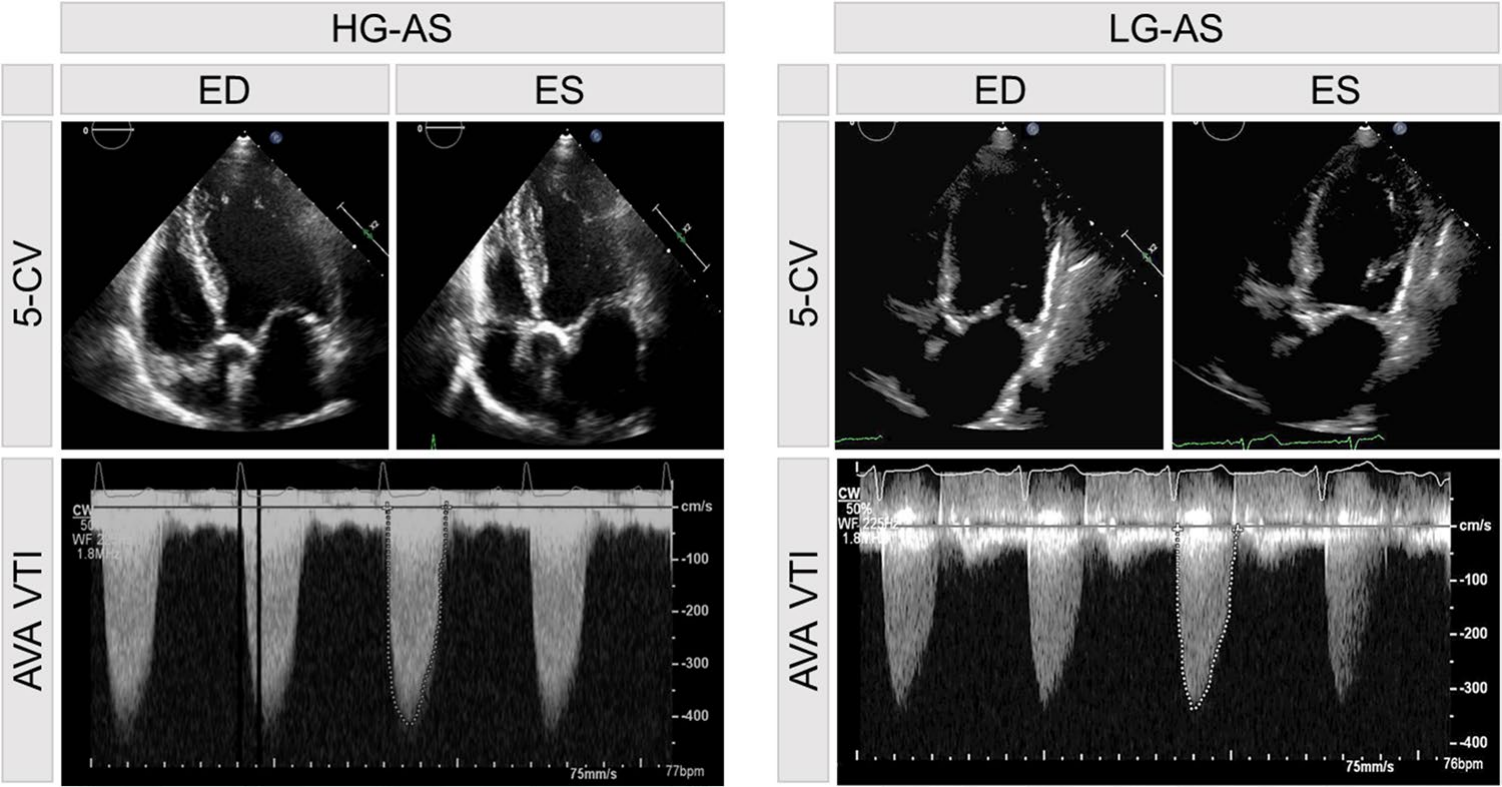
Echocardiographic images of patient with high-gradient (HG) and low-gradient (LG) aortic stenosis (AS), respectively. Pictures in the top showing a 5-chamber view (5-CV) in end-diastole (ED) and end-systole (ES). The corresponding derived aortic valve area velocity time integral (AVA VTI) signal is shown below
